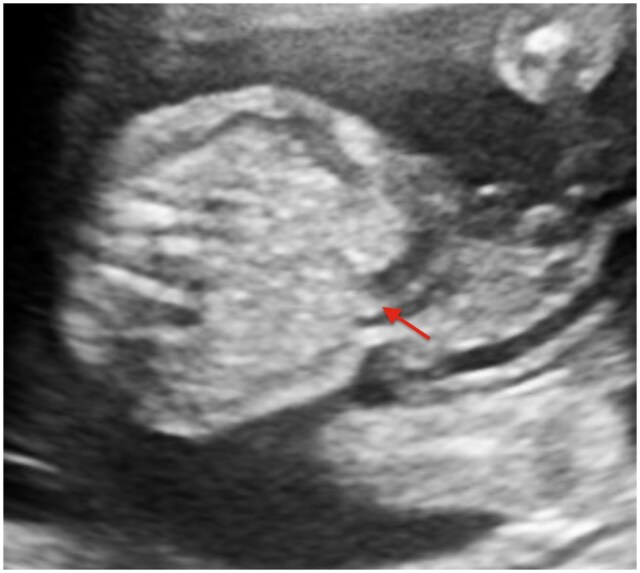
Ultrasound image of the fetal anterior abdominal wall demonstrating skin-covered herniation of bowel loops through a narrow umbilical defect with normal cord insertion (red arrow). The absence of bowel dilatation or abnormal echogenicity suggests preserved bowel viability.
